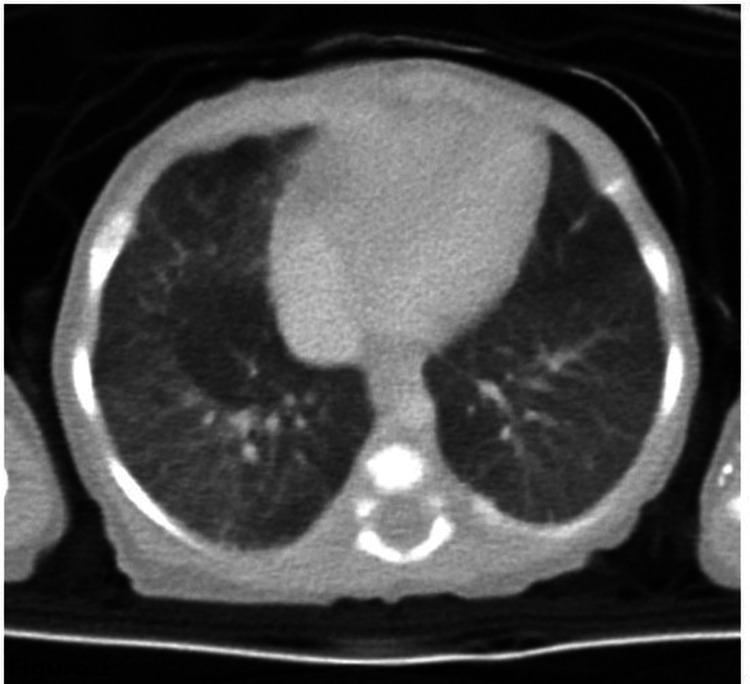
Axial high-resolution CT image at 27 days of life The image demonstrates diffuse mosaic attenuation in both lungs, possibly attributable to small airway disease.
